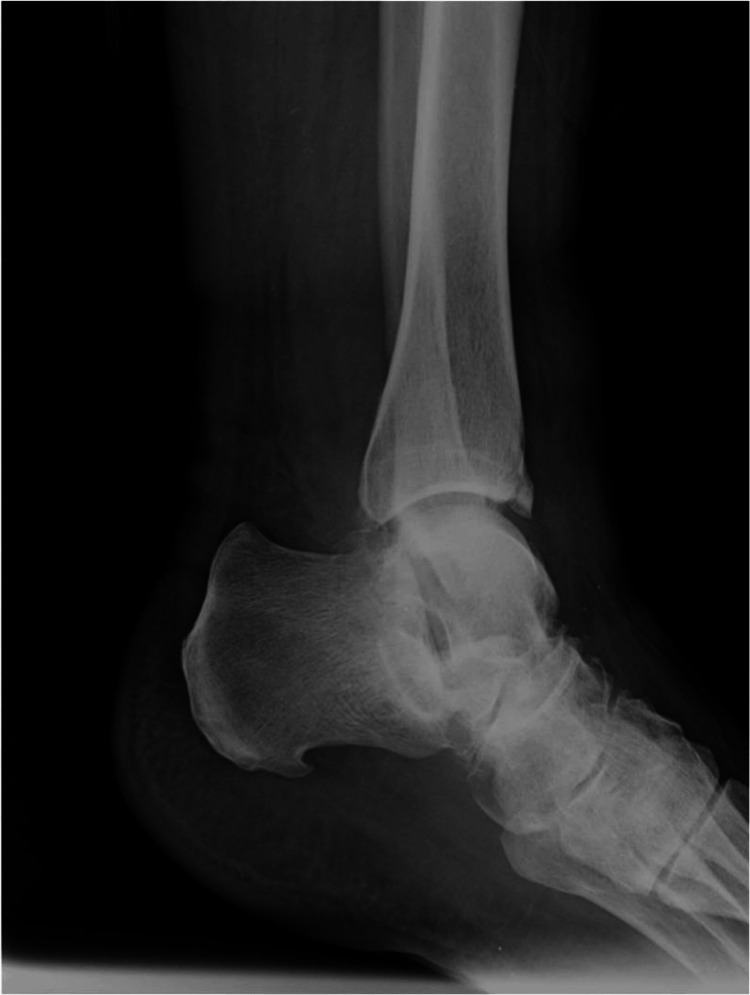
Preoperative lateral ankle X-ray
